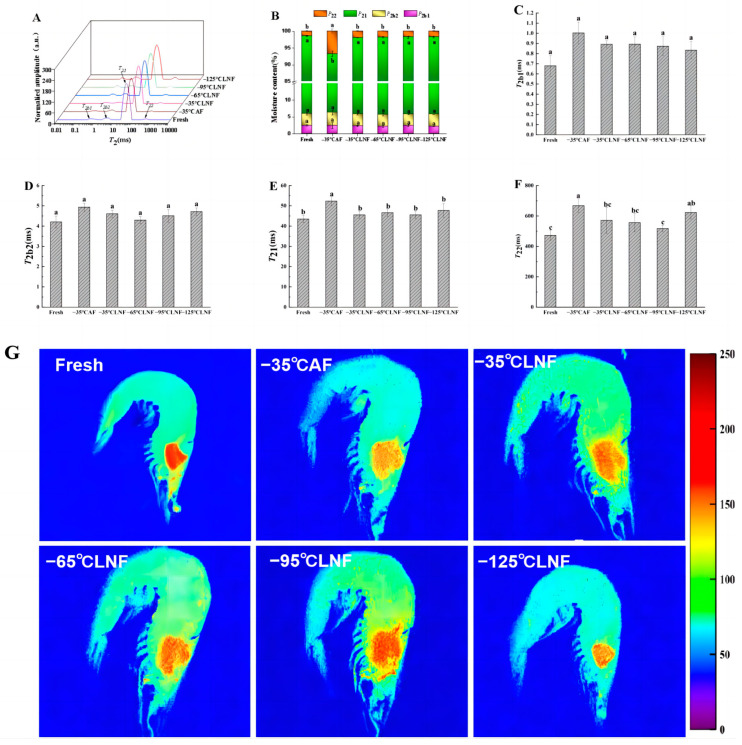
Changes in water properties in vannamei shrimp muscle under different frozen treatments. *T*_2_ relaxation times (A); different types of water content (%) (B); *T*_2b1_ relaxation times (C); *T*_2b2_ relaxation times (D); *T*_21_ relaxation times (E); *T*_22_ relaxation times (F); false-color images obtained via *T*_2_-weighted magnetic resonance imaging (G). The velocity bar right shows the correspondence between proton signal intensity and color, the weakening of the proton intensity corresponds to a gradual reddening of the colour of the bands. *P*_2b1_, *P*_2b2_, *P*_21_, and *P*_22_ represent the content of strongly bound water, weakly bound water, immobilized water, and free water, respectively. Different letters in the same index indicate a significant difference (*p* < 0.05).
